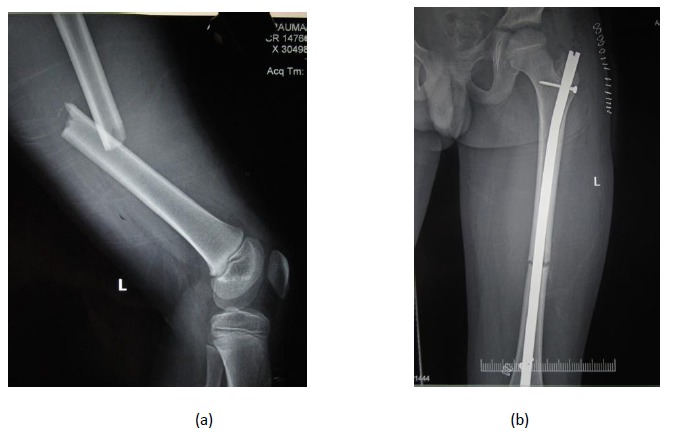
Pre-operative and post-operative radiographs of an 11 year old male with femoral shaft fracture treated by a trochanteric entry rigid intramedullary nail.
